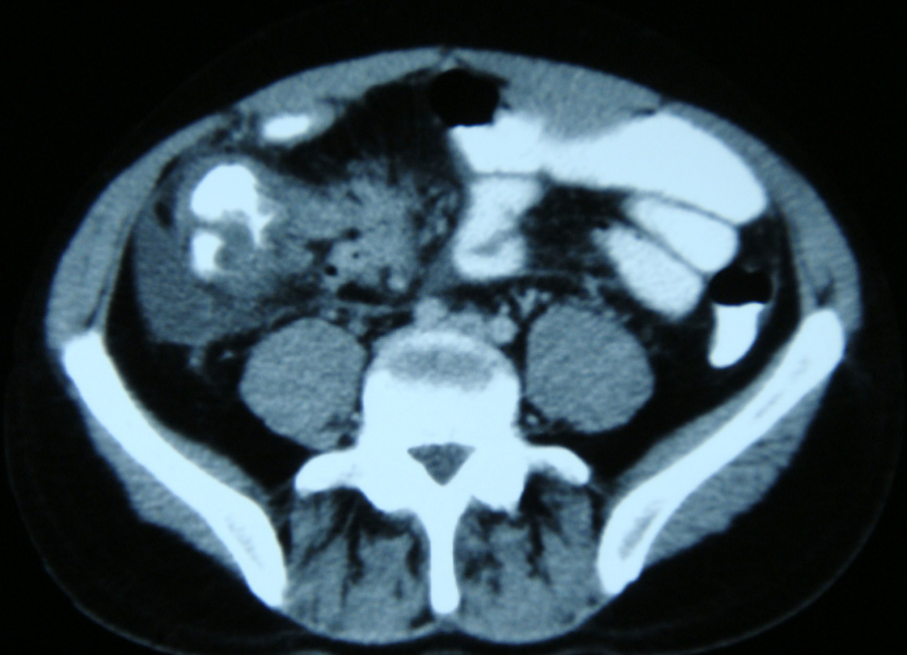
Contrast-enhanced abdominal computed tomography showing the cecal mass.
